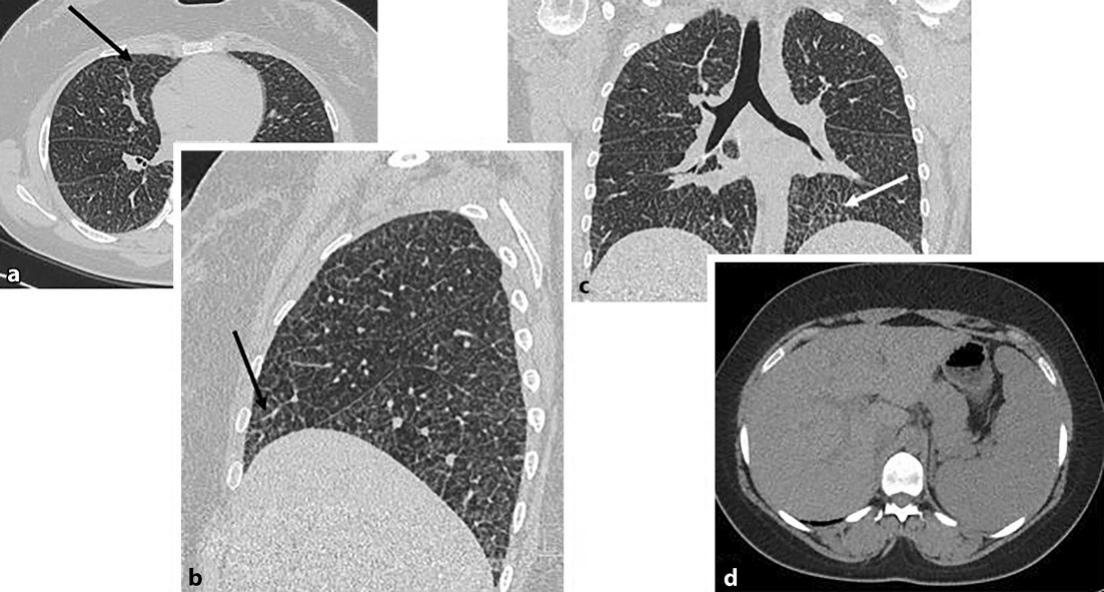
Morbus Pompe, eine seltene, erbliche Stoffwechselerkrankung, die durch einen Mangel des Enzyms saure Alpha-Glukosidase zu einer pathologischen Akkumulation von Glykogen in den Lysosomen führt. Sie äußert sich vorwiegend mit kardialer, muskuloskeletaler und hepatischer Beteiligung. Die axiale CT-Thorax auf Höhe der Lungenmittel- und Unterlappen (a) sowie die sagittale (b) und koronare (c) Rekonstruktion zeigen eine deutliche Verdickung der Interlobär- und Interlobulärsepten (*schwarze* und *weiße*
